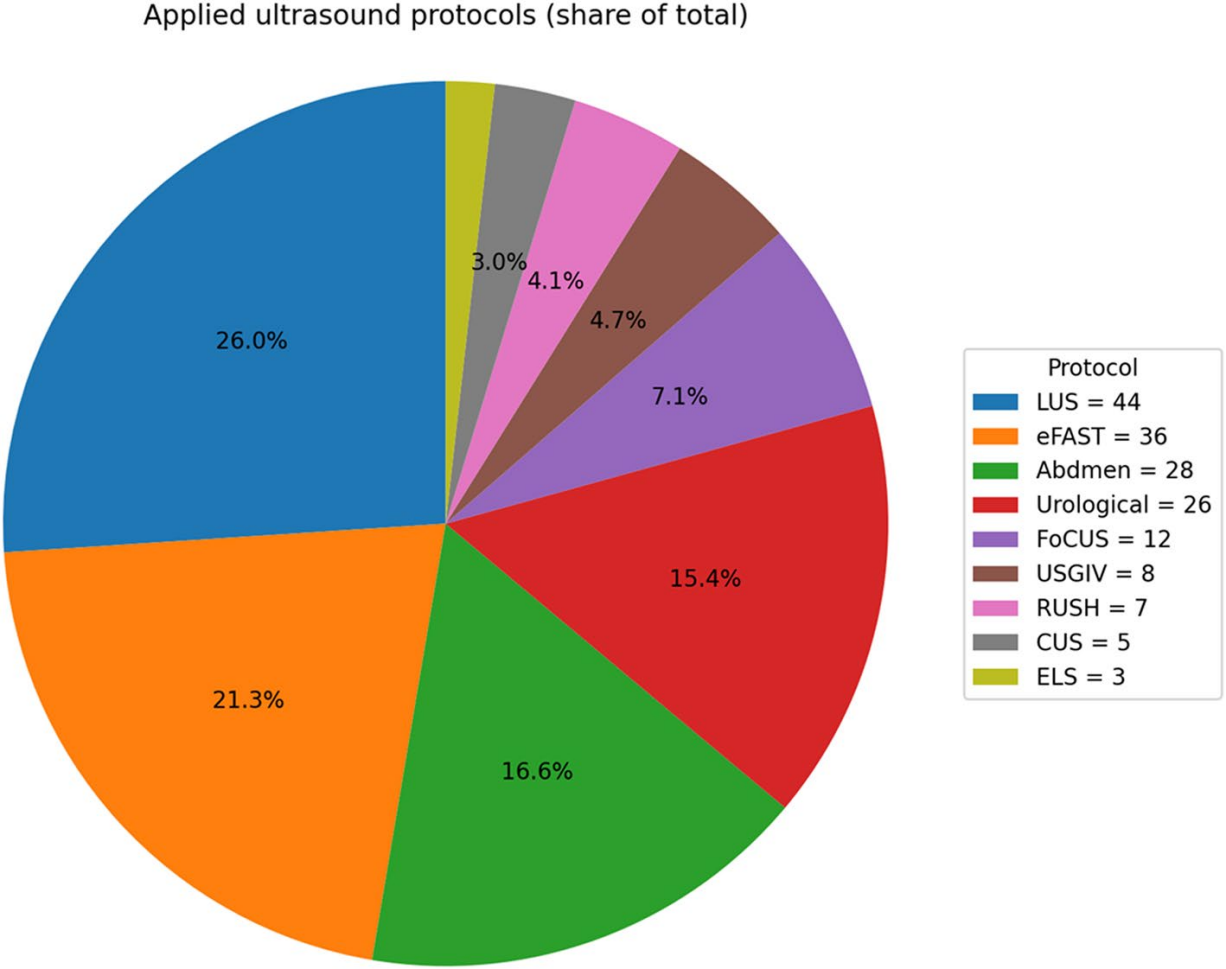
Distribution of applied prehospital ultrasound protocols. Lung ultrasound (LUS), Extended Focused Assessment with Sonography for Trauma (eFAST), Focused Cardiac Ultrasound (FoCUS), Rapid Ultrasound in Shock and Hypotension (RUSH), ultrasound-guided peripheral intravenous access (USGIV), and echocardiography in advanced life support (ELS). Values are shown as percentages of all performed examinations and absolute numbers
